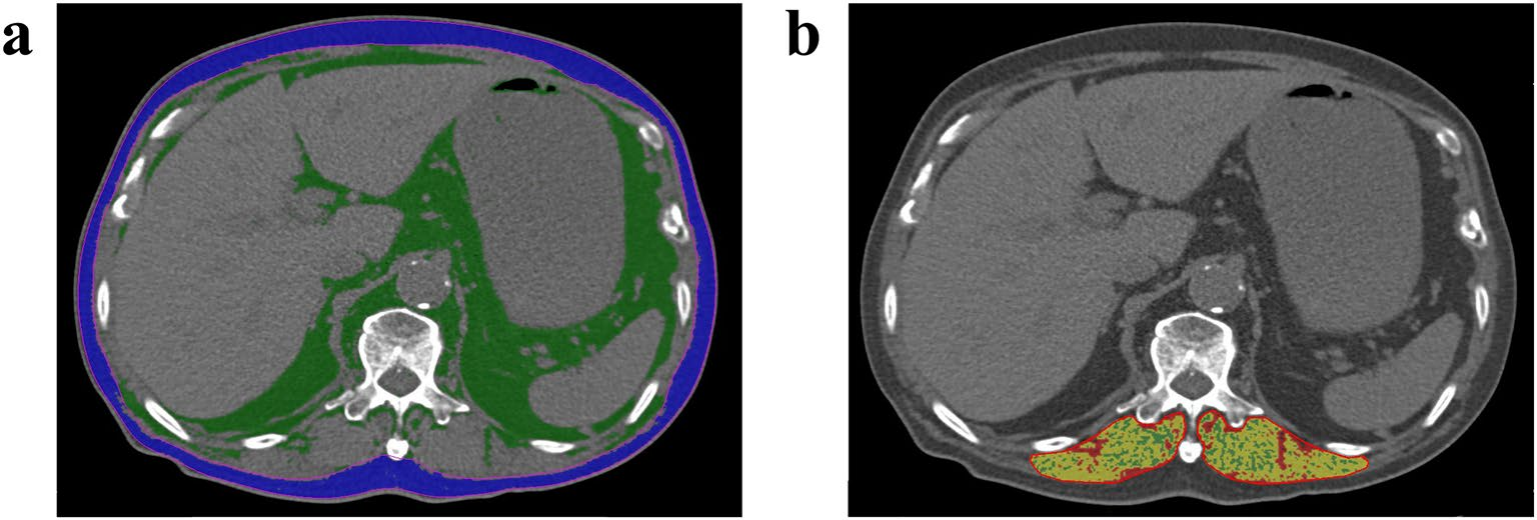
Computed tomography images show how body compositions are measured. (a) An image measuring the cross-sectional area of subcutaneous fat at L1 spine level (blue area). (b) An image measuring the cross-sectional area and Hounsfield unit of erector spinae muscles at L1 spine level (yellow area surrounded by red lines indicates muscle with a threshold of − 29 to + 150 HU; red and green areas indicate intermuscular fat with − 200 to − 100 HU and − 100 to − 30 HU, respectively).
